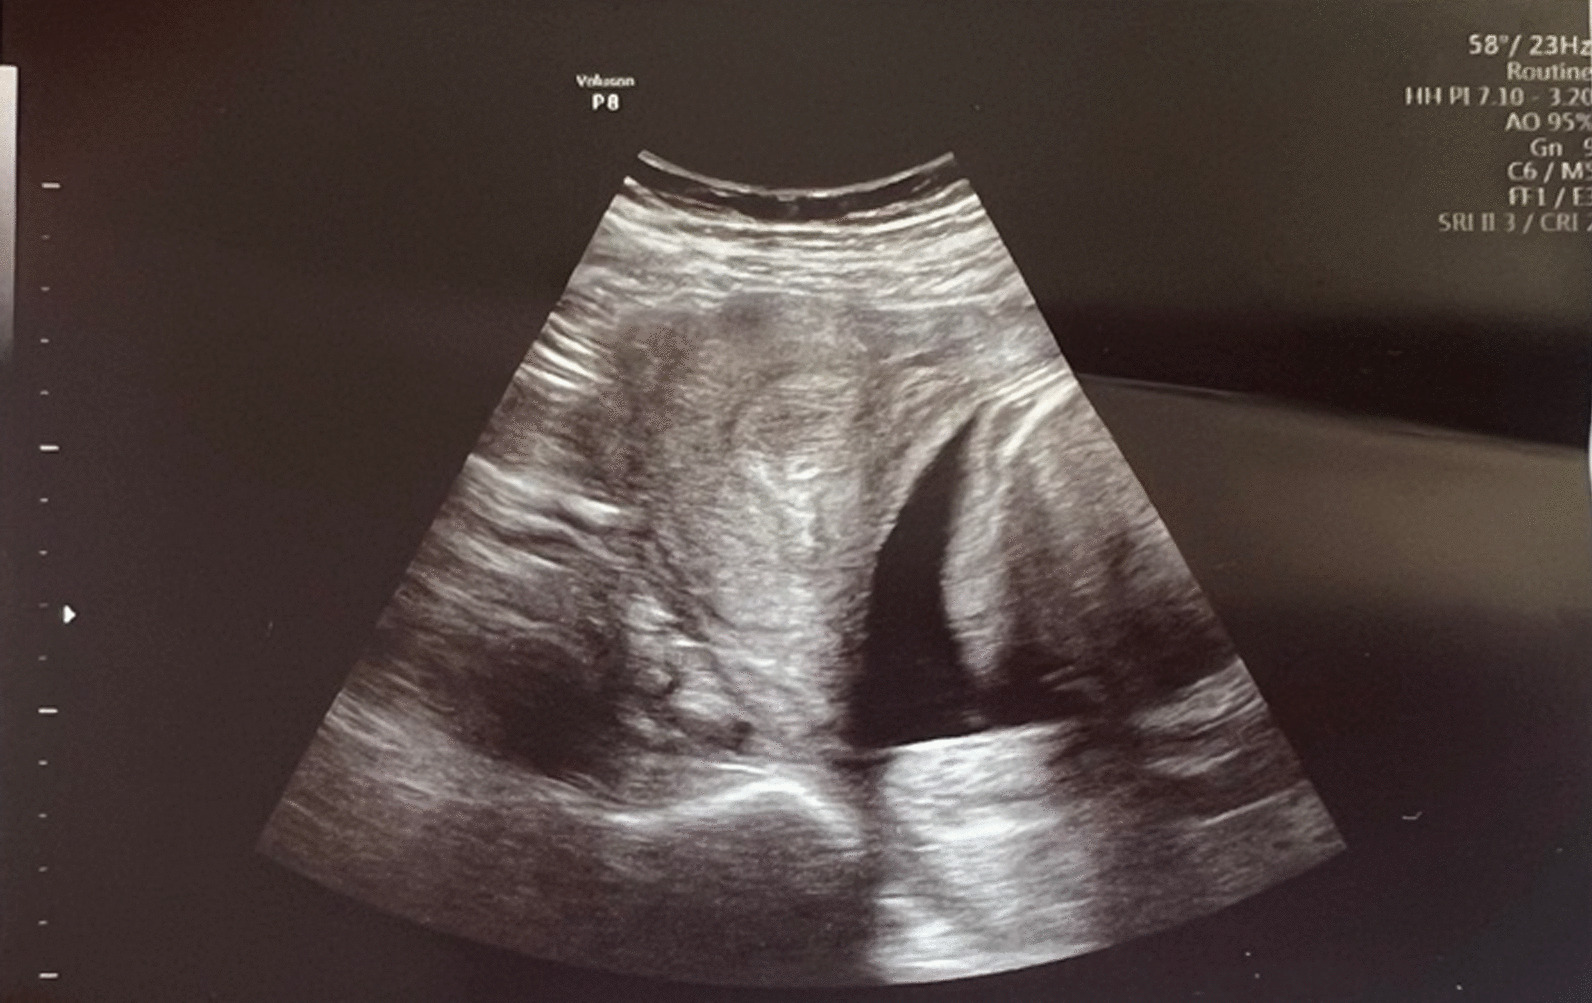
Ultrasound examination for control of contraction of the right uterine horn as well as exclusion of placental remnants after delivery of the first pregnancy
